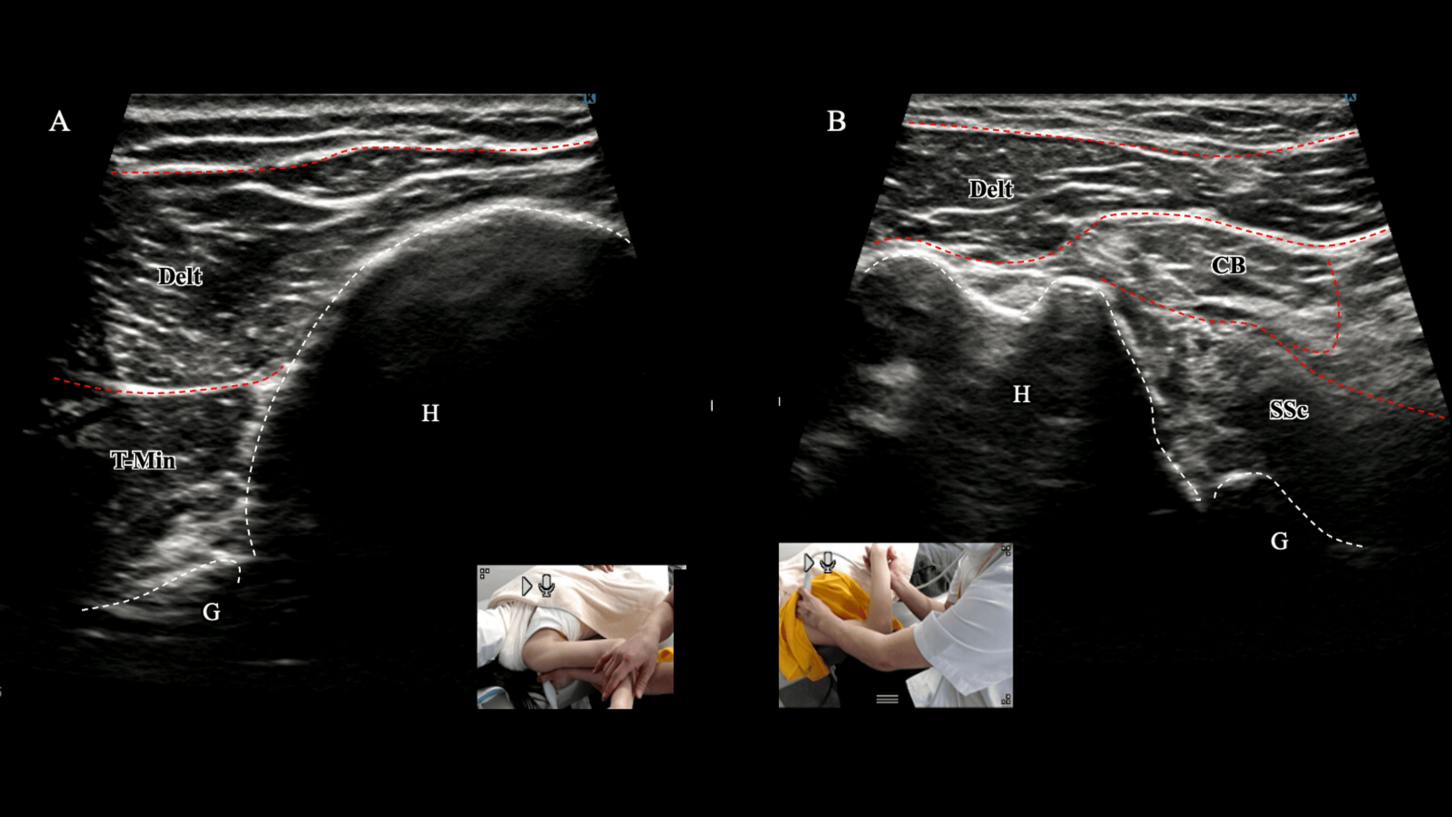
Post-training dynamics of the teres minor (A) and subscapularis (B). After repeated ultrasound-guided visual-feedback contraction exercises, the T-Min during external rotation (ER) shows smooth thickening/shortening without localized folding, and the SSc demonstrates greater end-range internal rotation (IR) excursion with improved awareness/selective activation. These changes paralleled the clinical improvements in ER/IR strength and movement apprehension. Delt, deltoid; T-Min, teres minor; SSc, subscapularis; CB, coracobrachialis; G, glenoid; H, humeral head
